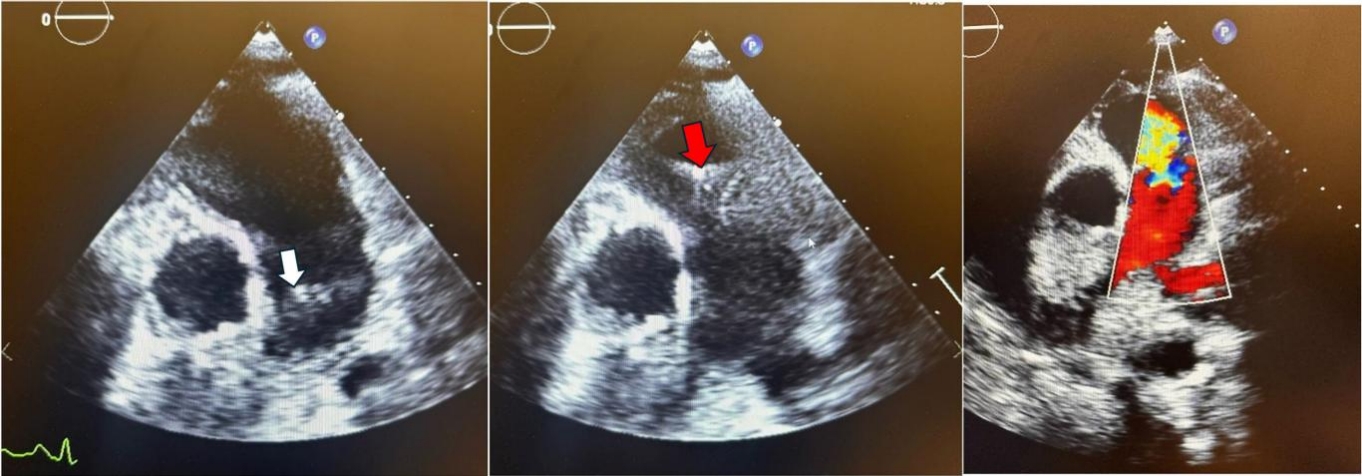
Parasternal short-axis echocardiogram with colour Doppler during the initial pulmonic valve infective endocarditis episode showing pulmonic valve vegetation during systole (white arrow), diastole (red arrow), and severe pulmonic regurgitation.
